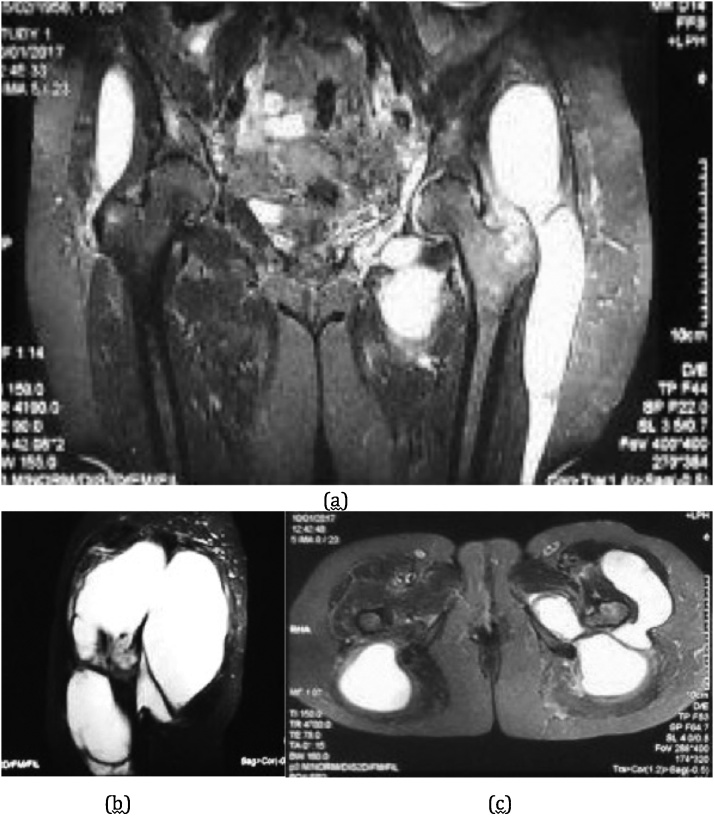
(a) Coronal T2-FS MRI images of pelvic and bilateral femur showing extension of the abscess distally around the greater trochanter beneath the gluteus maximus muscle and tensor fascia lata; (b) Sagittal T2-FS MRI images centered around the greater trochanter; (c) Axial T2-FS MRI cut on the level of proximal femur just distal to the lesser trochanter showing pattern of abscess extension distally.
